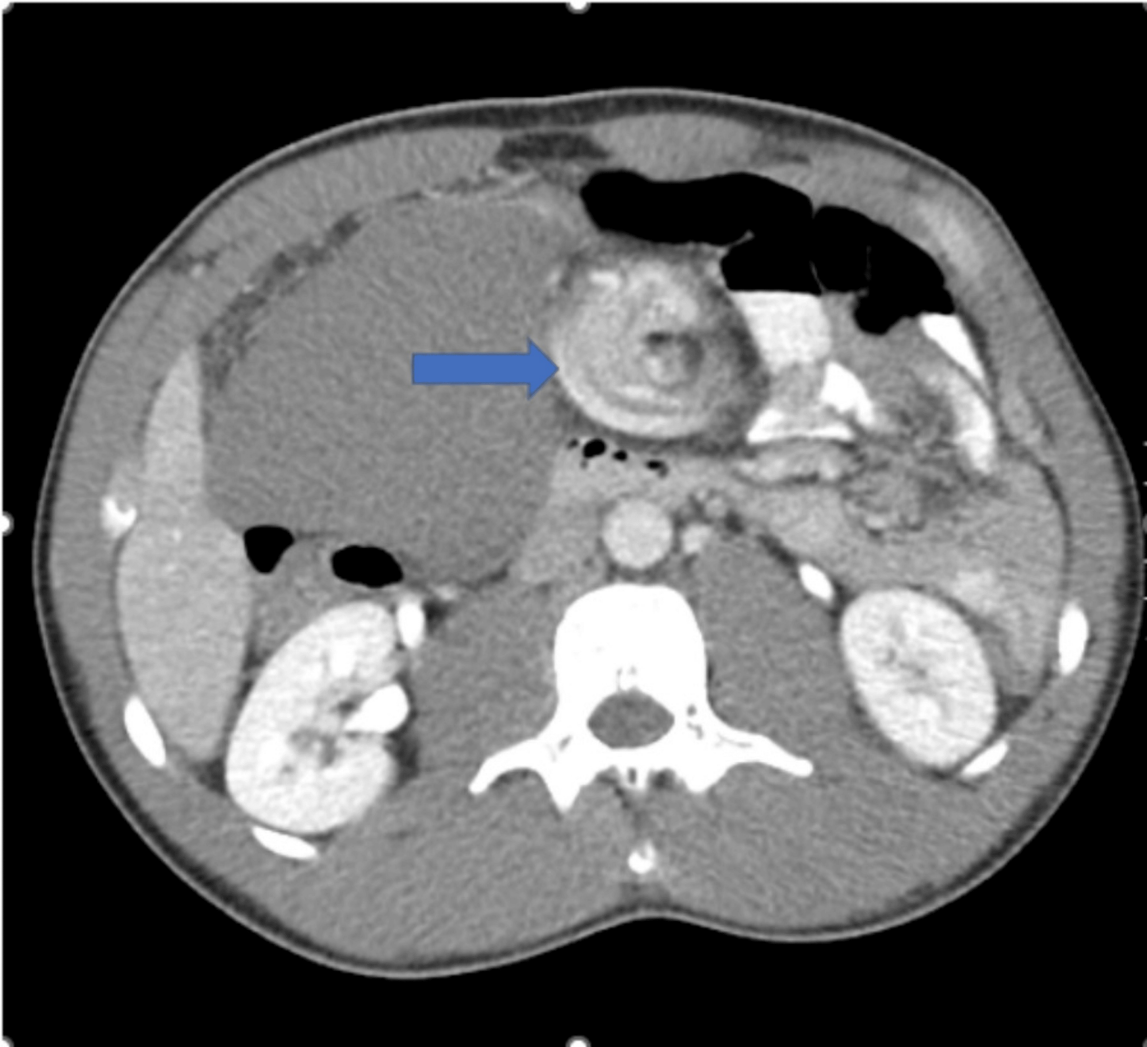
CT abdomen and pelvis, axial view, slice 36. Blue arrow representing twisting of the splenic pedicle.
